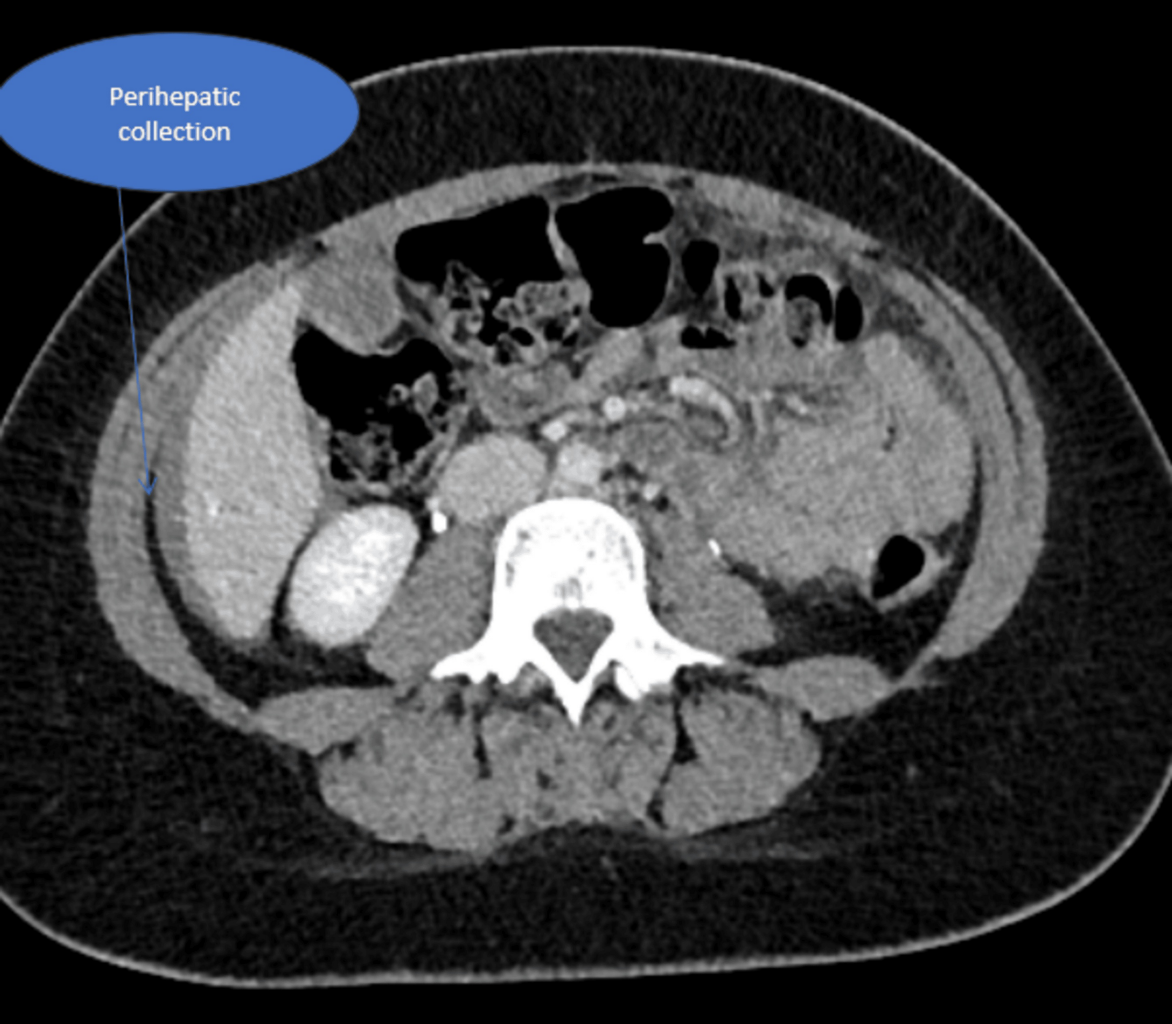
CT of the abdomen and pelvis shows bleeding around the liver
